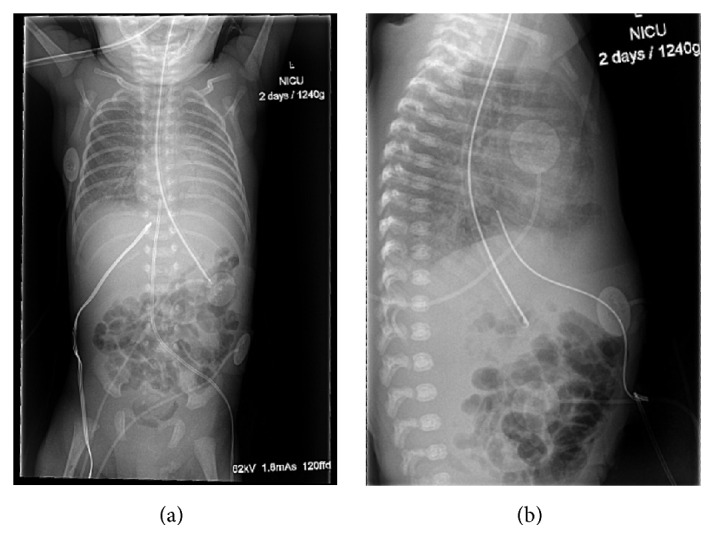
AP and lateral chest and abdomen X-rays taken after onset of SVT. The tip of the catheter is seen to have migrated into the right atrium.
